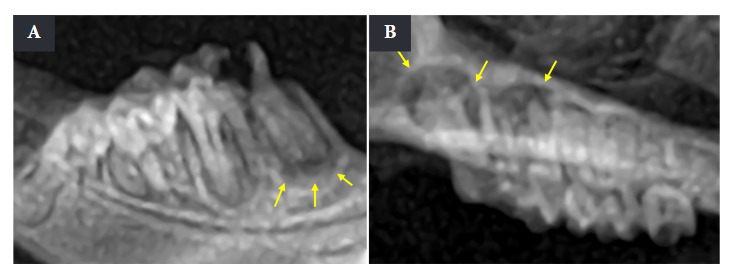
Radiographs of periapical lesions. A) Periapical lesion in the mandibular first molar. B) Periapical lesion in the maxillary first molar. The yellow arrows indicate the limits of each lesion.
